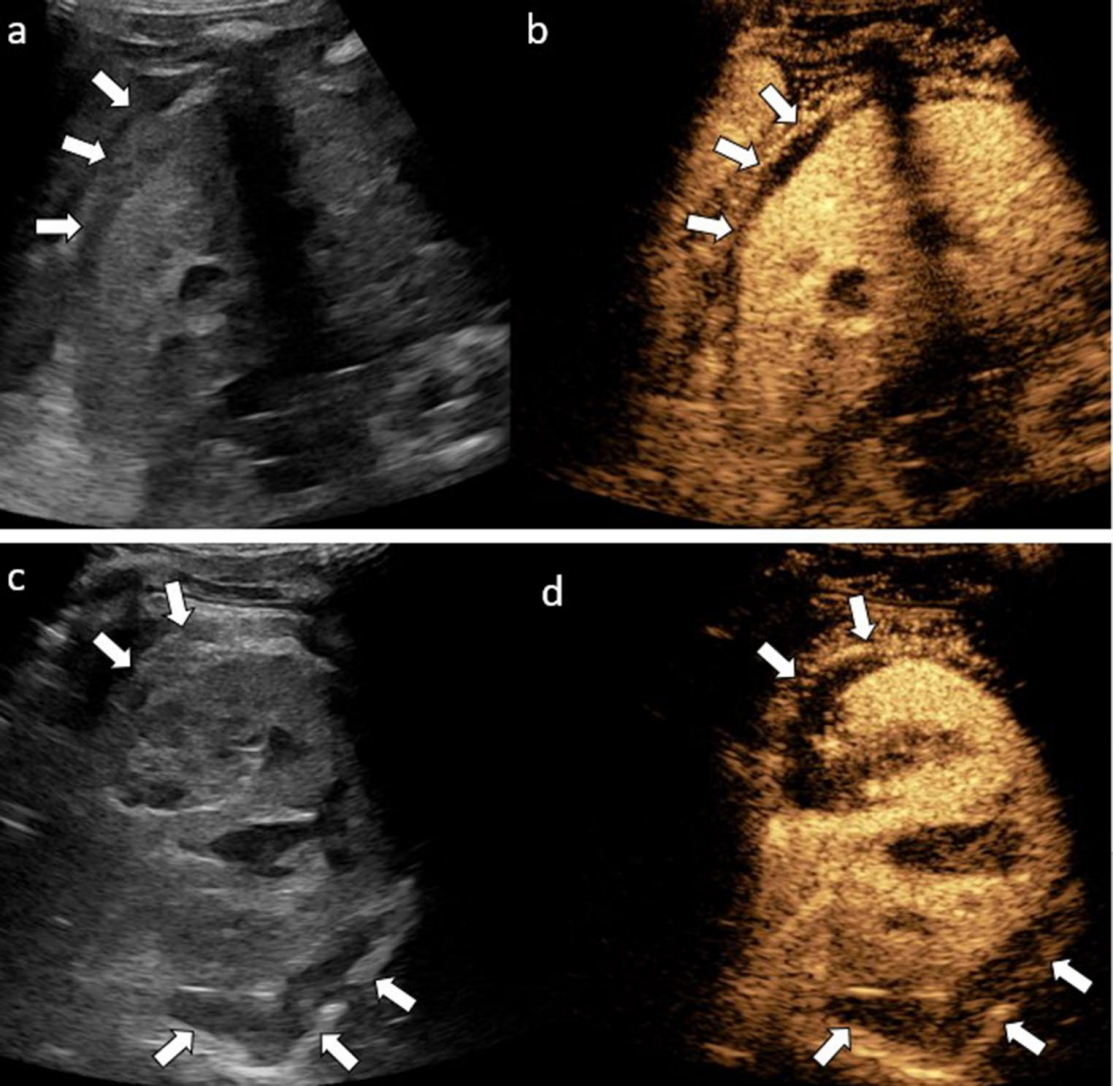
Subcapsular renal hematoma in a pediatric patient. (a, b) Sagittal and (c, d) transverse contrast-enhanced ultrasound (CEUS) images of the left kidney with a dual display of gray-scale (left) and contrast (right) modes. The gray-scale US images (a, c) reveal a hypoechoic subcapsular fluid collection (arrows) around the superior pole of the left kidney. CEUS images (b, d) demonstrate a large non-enhancing subcapsular hematoma (arrows)
